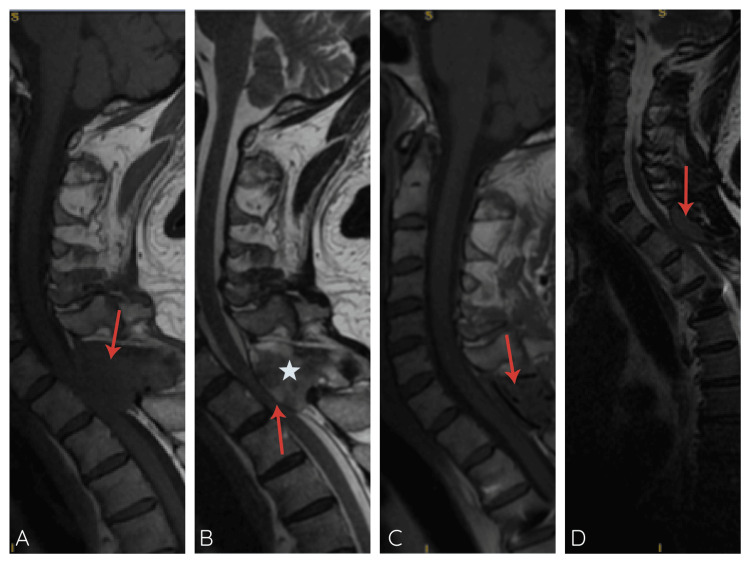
Magnetic resonance imaging (MRI) of the cervical spine (A) T1-weighted MRI sequence showing a lesion with signal intensity like that of adjacent soft tissues (red arrow). (B) T2-weighted MRI sequence revealing a heterogeneous mass involving the posterior paraspinal tissues (star), with contiguous epidural extension and spinal cord compression (red arrow). (C) Post-chemotherapy T1-weighted sequence, demonstrating a partial treatment response (red arrow). (D) Follow-up T1-weighted sequence showing an oval soft-tissue mass with slightly hyperintense signal and epidural extension (red arrow), consistent with local relapse.
